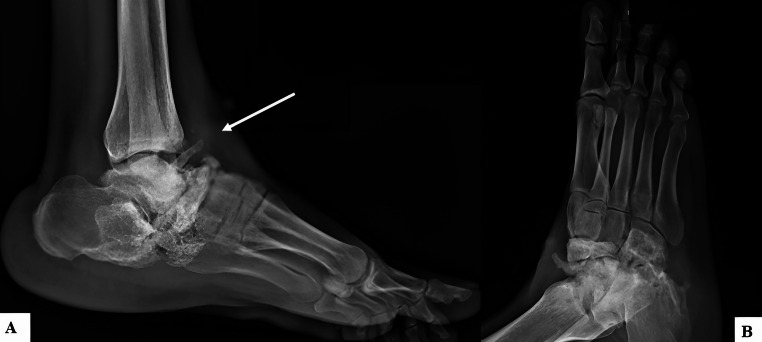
Ankle and foot X-ray of an asylum seeker victim of gunshot wound. Xray in anteroposterior (A) and lateral (B) projection shows foreign body retention due to gunshot wounds in the subcutaneous tissue anterior to the Talus bone (white arrow)
